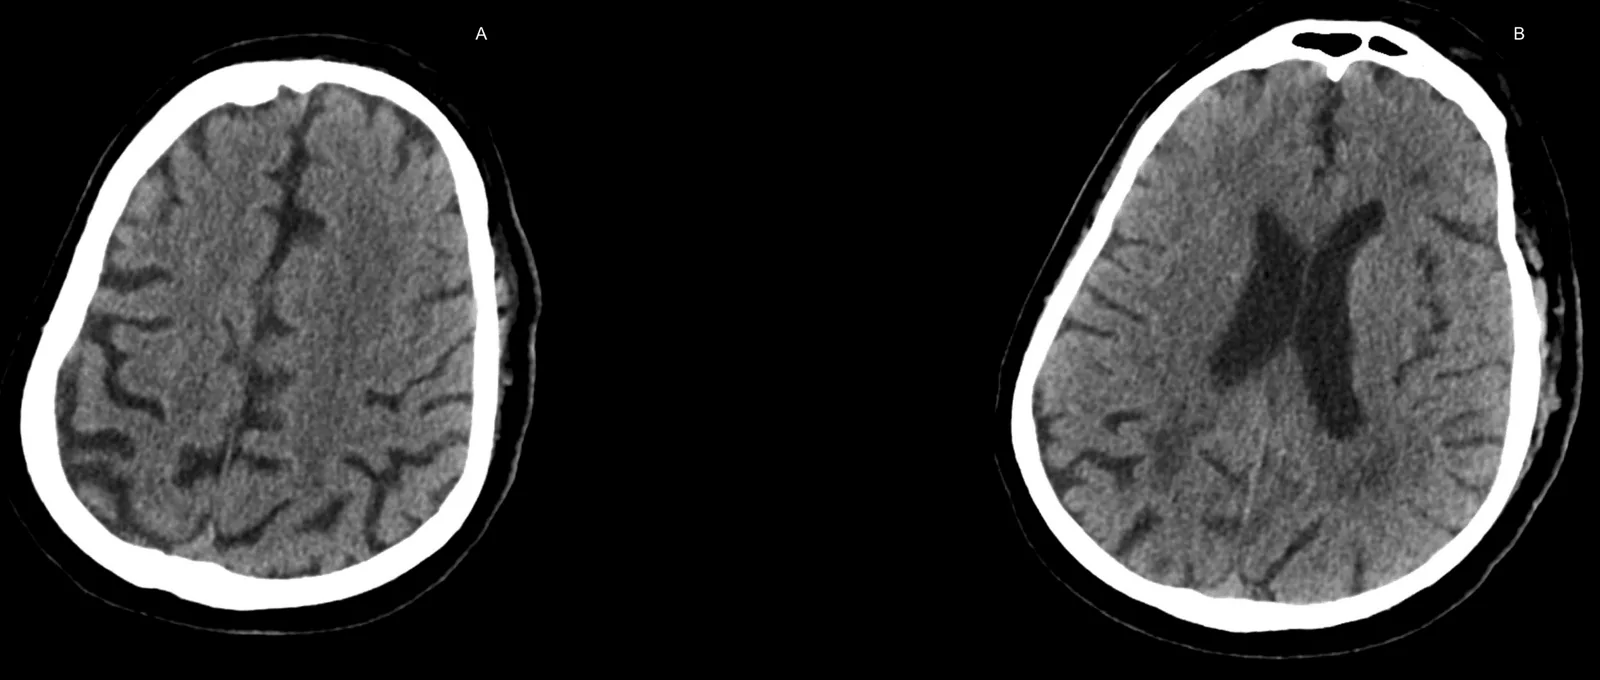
Non-contrast head CT (first admission) A, B: sequential axial non-contrast CT images demonstrating no acute intracranial hemorrhage, mass effect, midline shift, or established large-territory infarct.
